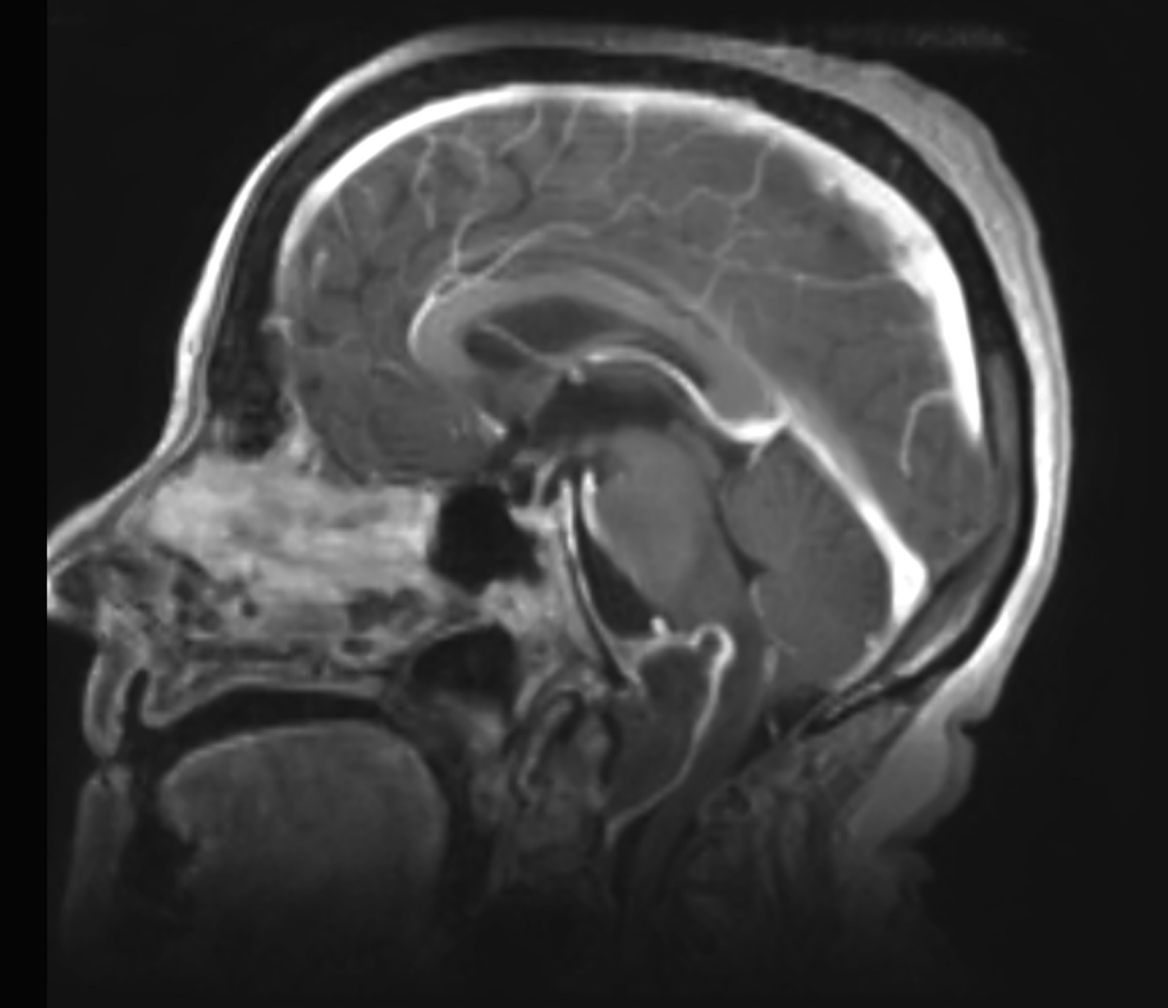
September Sagittal MRI Enhancing mass
